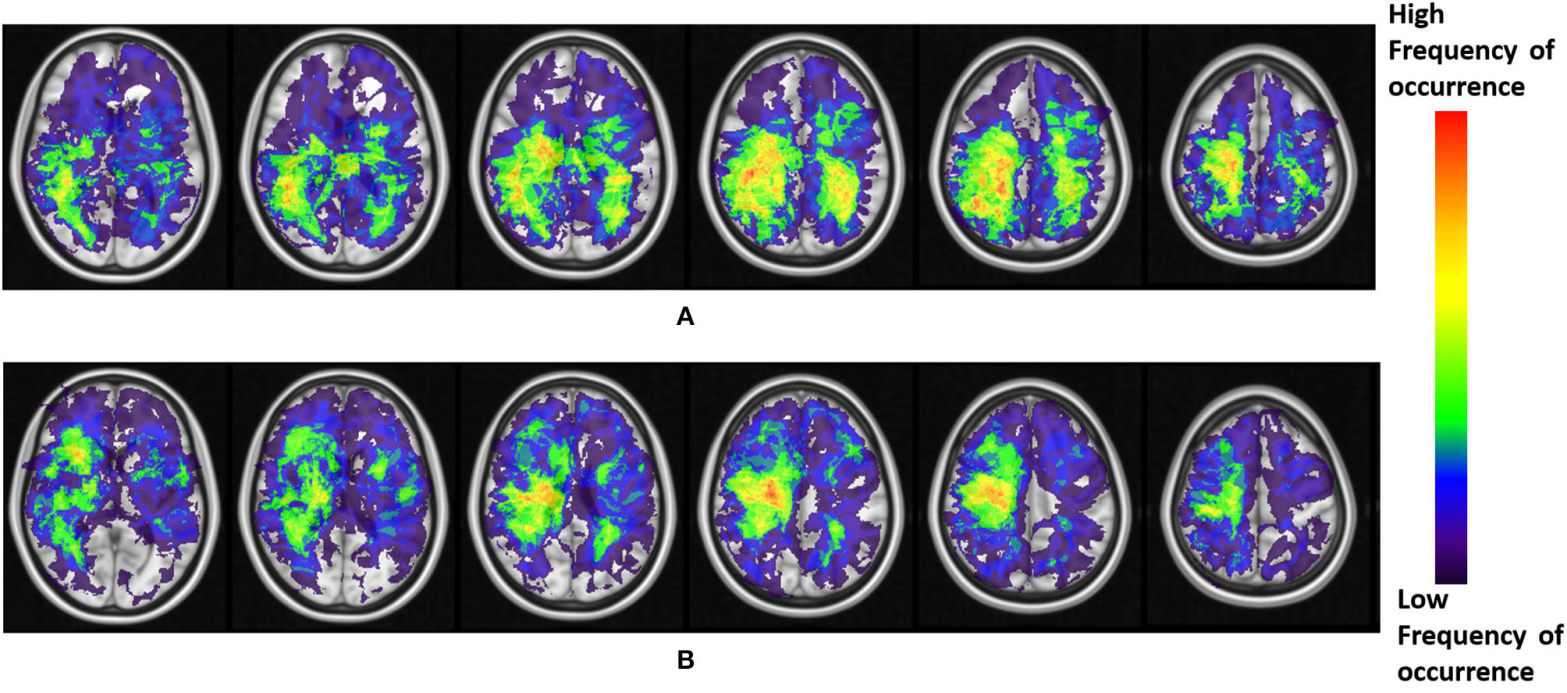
(A) Frequency maps of tumor occurrence for peri-lesional T2/FLAIR hyperintensities in tumor recurrence of pre-treatment scans, where lobar prominence is present in the parietal lobe of both hemispheres. (B) Frequency maps of tumor occurrence for peri-lesional T2/FLAIR hyperintensities in pseudo-progression, where a multi-focal distribution is present in the frontal lobe, temporal lobe, insula, and putamen of the right hemisphere.
